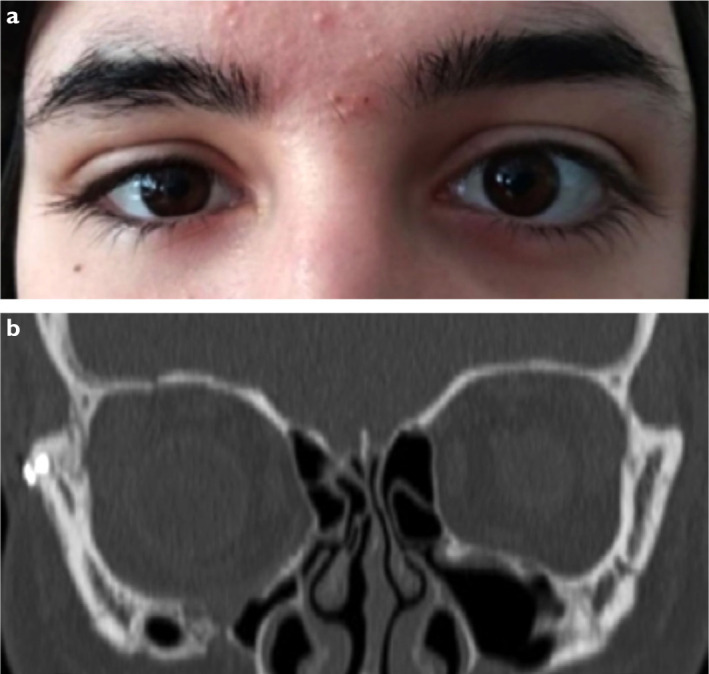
Patient at 14 weeks follow-up with improved (a) hypoglobus and enophthalmos (b) CT scan at 14 weeks follow up.
